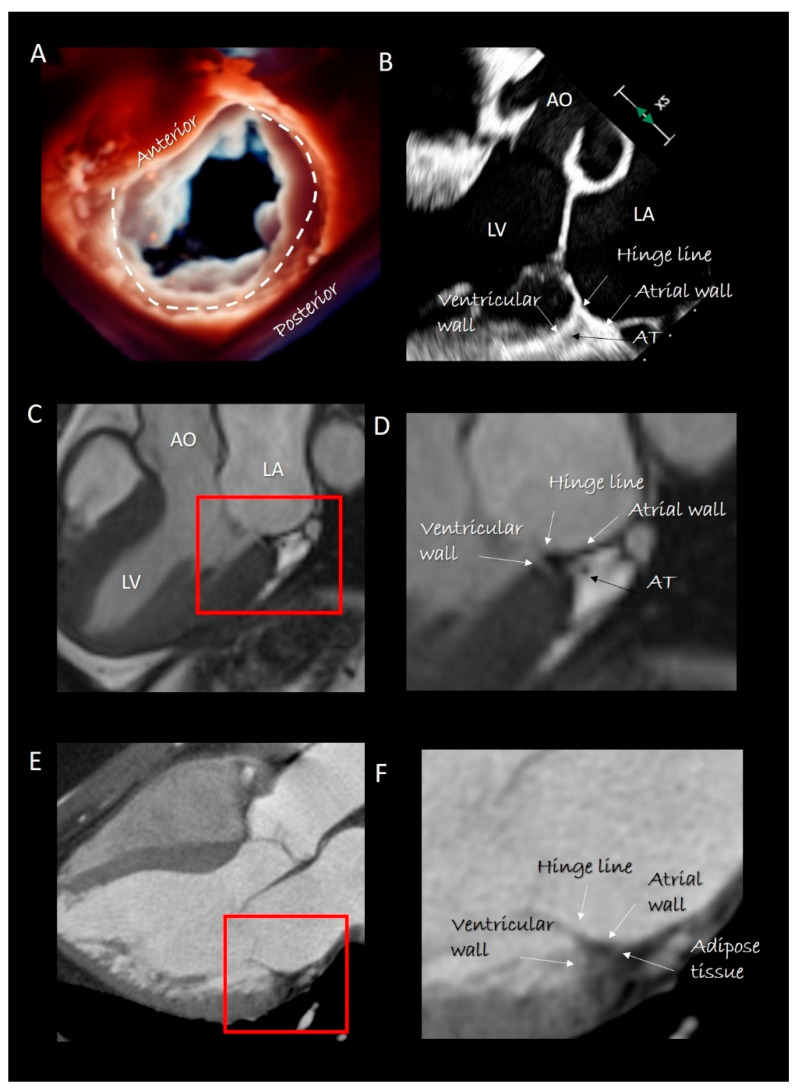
(A) 3D transesophageal echocardiography (TEE) still frame image of mitral valve forms an overhead perspective with a specific rendering algorithm that permits a movable source of light. With a source of light behind the valve, the two components of mitral annulus are well visible (the curved dotted line marks the posterior segment). (B) 2D TEE cross-section long-axis view showing the four components of posterior segment of annulus. The adipose tissue (AT) has a different texture compared with surrounding tissues. (C) CMR cross-section long-axis view. (D) Magnified image of the structures inside the red square in C showing the four components of posterior segment. The AT can be distinguished because the signal is much stronger (white in color) than that of surrounding structures. (E) Computed tomography (CT) cross-section long-axis view. (F) Magnified image of the structures inside the red square in E showing the four components of the posterior segment. As AT is more hypodense to x-ray, it appears as an area that is darker than the surrounding structures. AO = aorta; LV = left ventricle; LA = left atrium.
